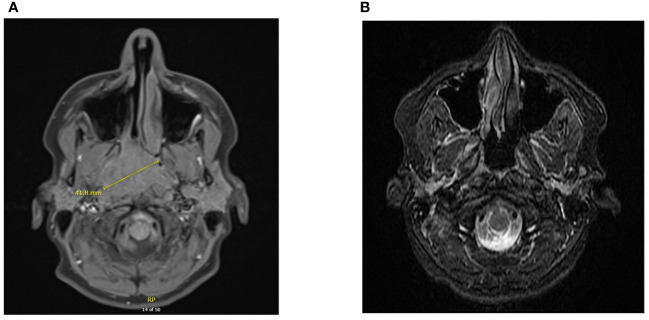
Diagnostic imaging of NPC at primary site. CT scan demonstrating a large mass at the right nasopharynx upon diagnosis (A). MRI of nasopharynx demonstrating complete response following chemoradiation and adjuvant chemotherapy (B).
